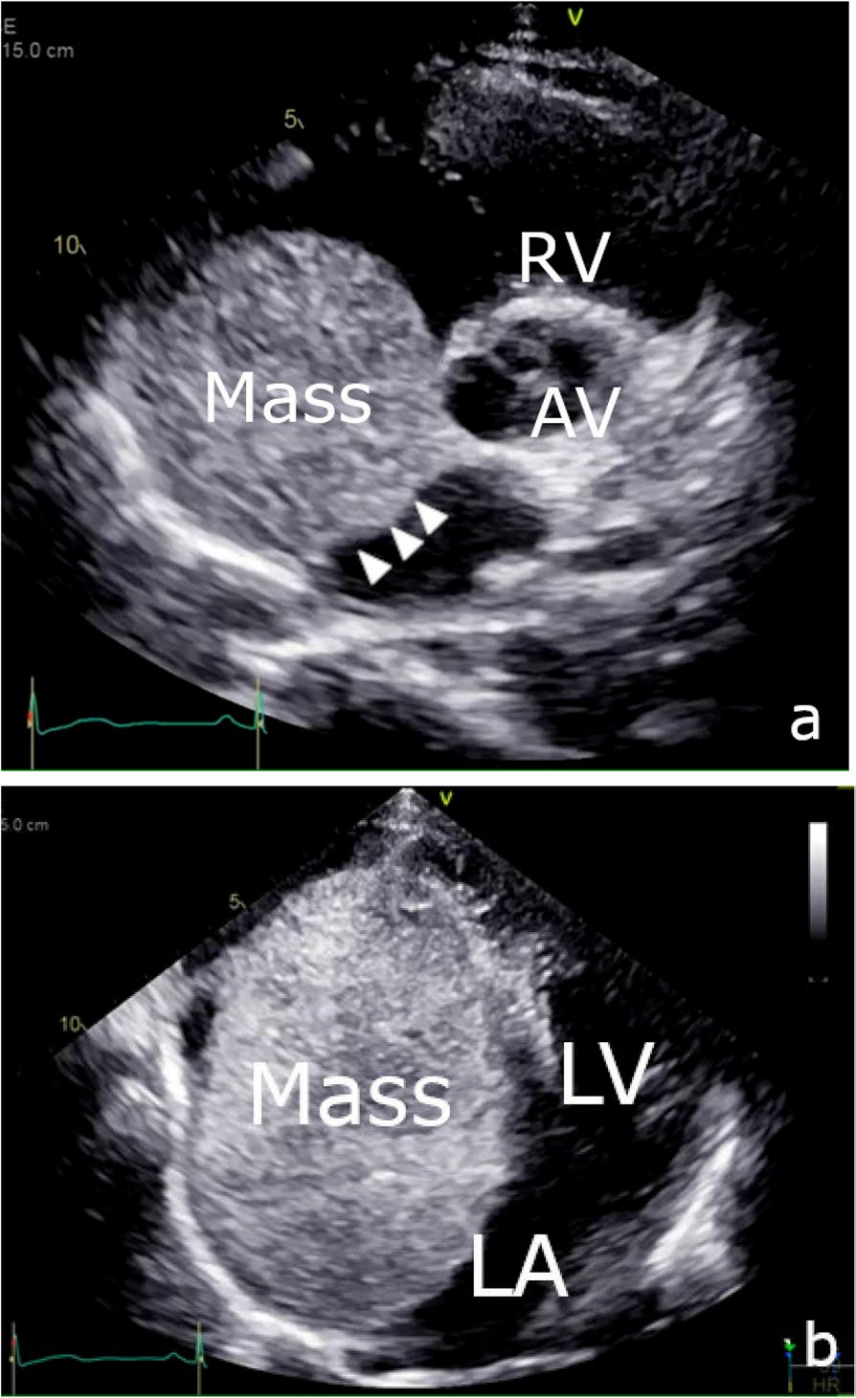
Preoperative transthoracic echocardiography. A short-axis view of aortic valve level (a) showing the mass with a smooth surface attaching the septal wall (white arrowhead) and a four-chamber view (b) showing the mass compressing both heart ventricles. The mass measures 115 × 92 mm in size without ultrasonic signals of blood flow. AV, aortic valve; LA, left atrium; LV, left ventricle; RA, right atrium; RV, right ventricle
